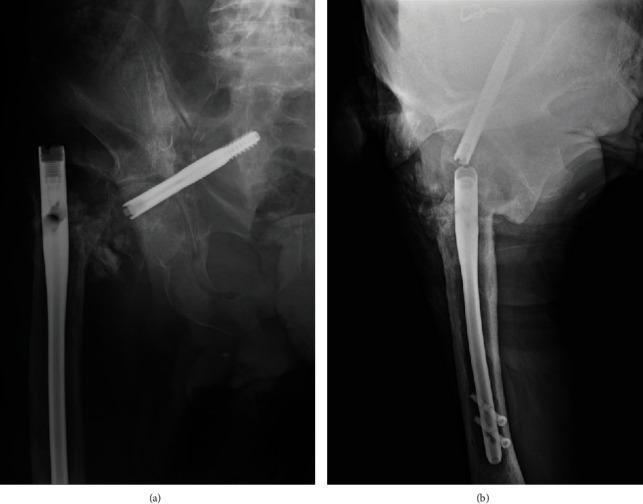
Radiographs showing redisplacement of the fracture and intrapelvic migration of the lag screw through the femoral head and the medial wall of the acetabulum.
